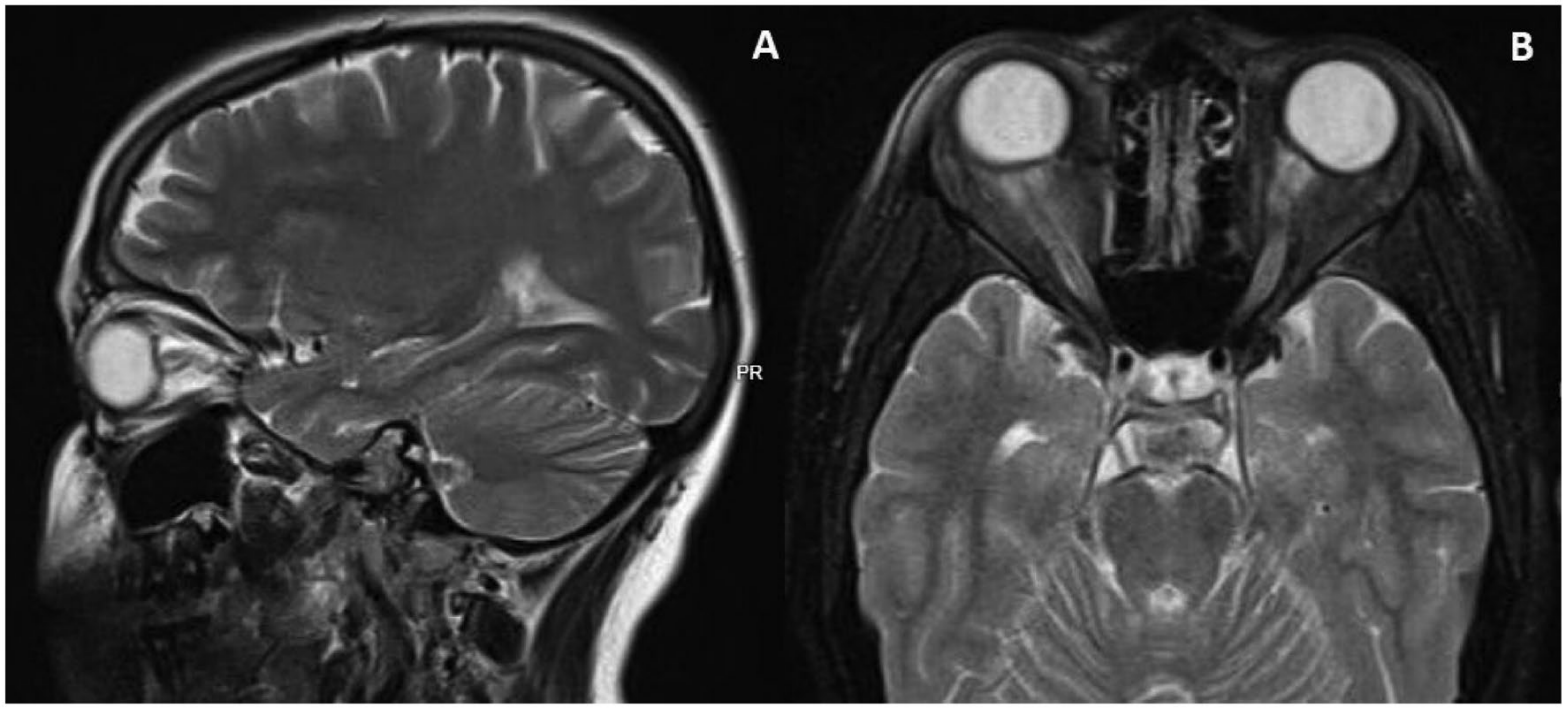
Brain MRI of Case 1 during her first admission. (A) Sagittal MRI showing widening of Optic Nerve subarachnoid space. (B) Axial MRI showing flattening of posterior aspect of the globe.
